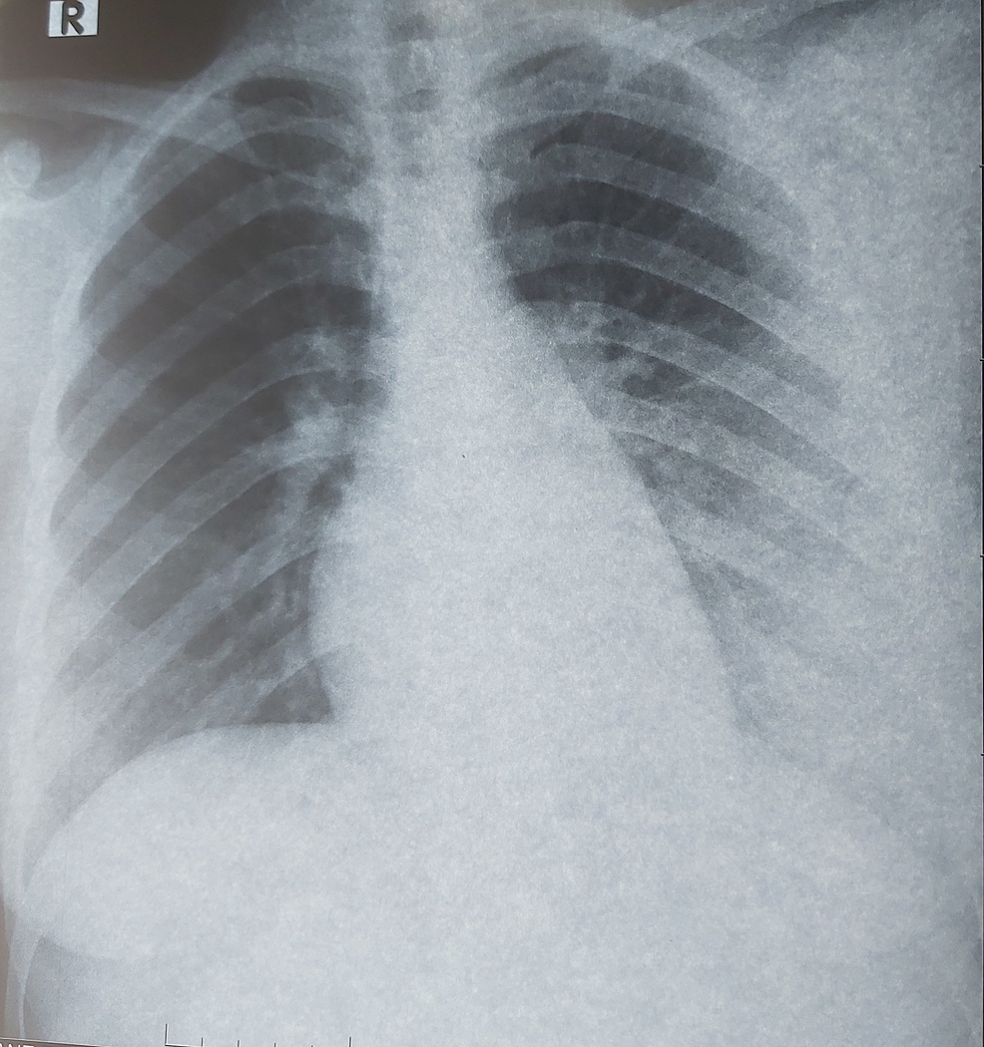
Control chest X-ray, showing improvement of lung involvement under corticosteroid therapy
